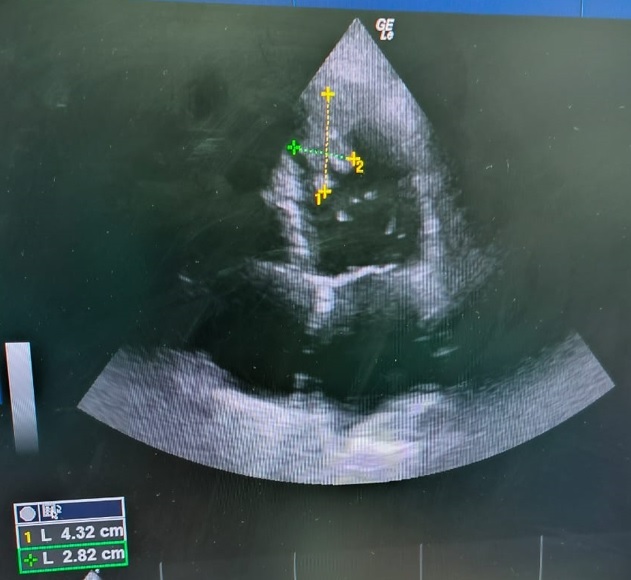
Transthoracic echocardiography; a four-chamber view of the heart after the bilateral mechanical thrombectomy and fasciotomy showing a pedunculated mass (thrombus) measuring 4.32*2.82 cm.
